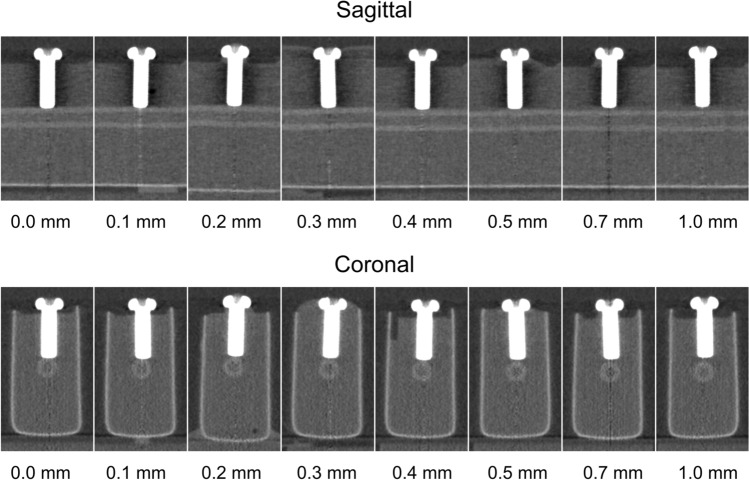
Sagittal and coronal CBCT images at each screw–tube distance. Images were uniformly cropped to a consistent region of interest without rotation or nonlinear manipulation. CBCT: cone-beam computed tomography
